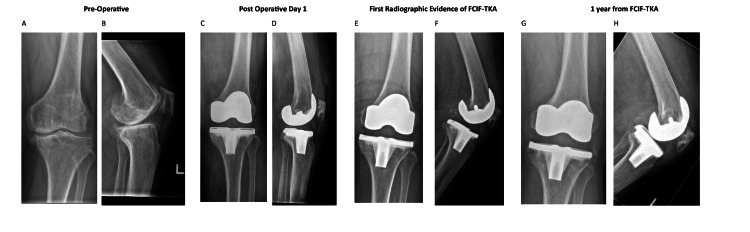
Patient 3 AP and lateral knee FCIF-TKA radiographs A: preoperative AP film, B: preoperative lateral film, C: postoperative day 1 AP film, D: postoperative day 1 lateral film, E: first radiographic evidence of FCIF-TKA AP film, F: first radiographic evidence of FCIF-TKA lateral film, G: one year from FCIF-TKA AP film, H: one year from FCIF-TKA lateral film AP: anterior-posterior, FCIF-TKA: femoral condyle insufficiency fractures following total knee arthroplasty
